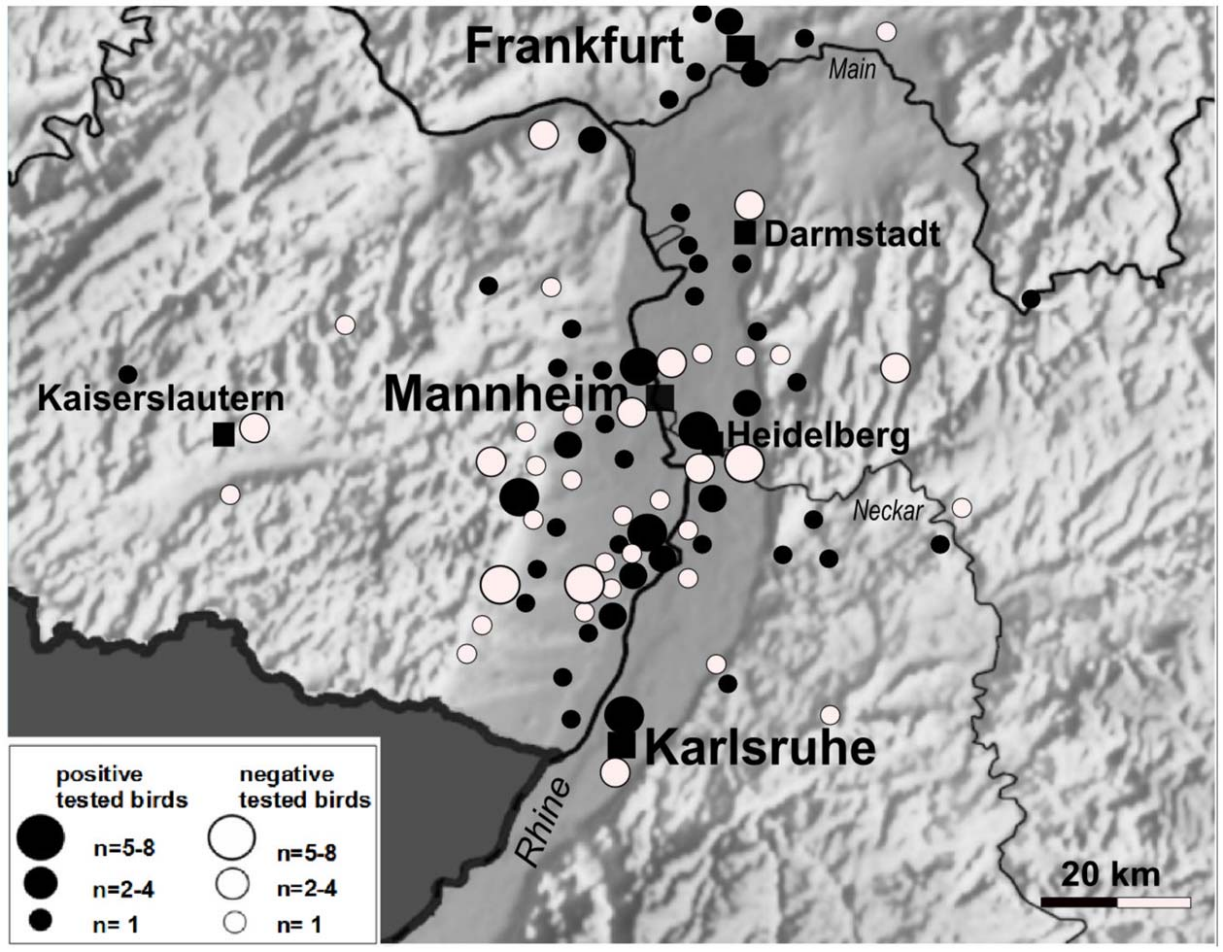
Spatial distribution of USUV RNA positive (n = 83) and negative (n = 61) tested birds found dead in southwest Germany. Black dot indicate birds tested positive for USUV RNA and white dot indicate USUV RNA negative birds. 79 birds were collected outside of region shown in the map.
